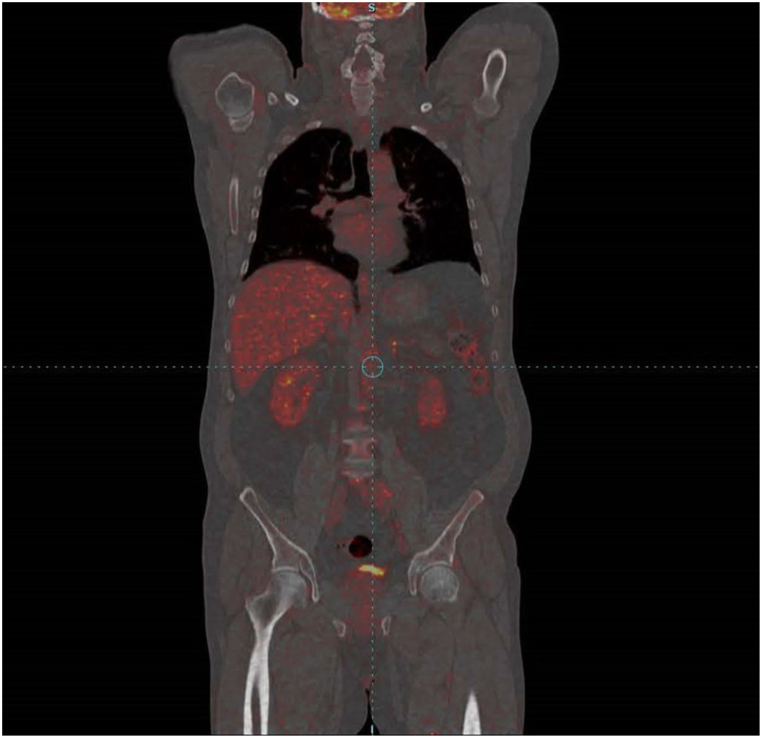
Positron emission tomography/computed tomography (PET/CT) revealed a complete metabolic response without signs of metabolically active disease.
